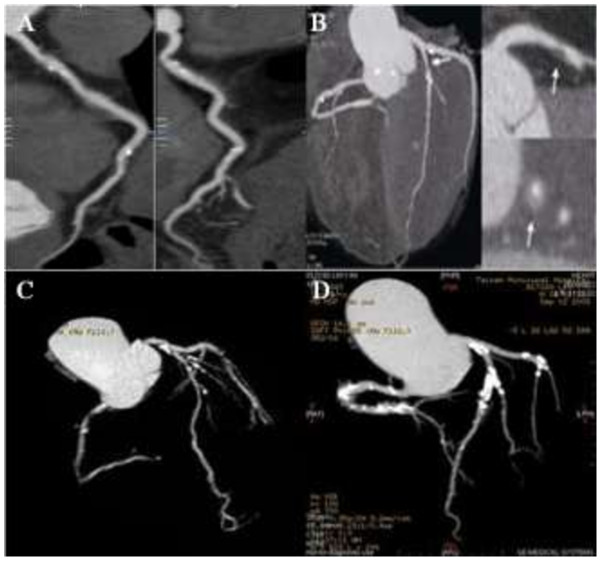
320-slice CT scans show the typical variable degree of CAC images among diabetic patients. (A): mild calcification, CACS = 89; (B): moderate calcification, CACS = 374; (C): severe calcification, CACS = 525; (D): extensive calcification, CACS = 1986.
